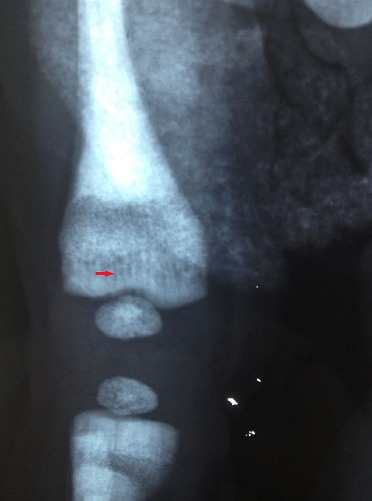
Right femur radiograph. Note metaphyseal modelling defects and characteristic lucent bands (arrow)
